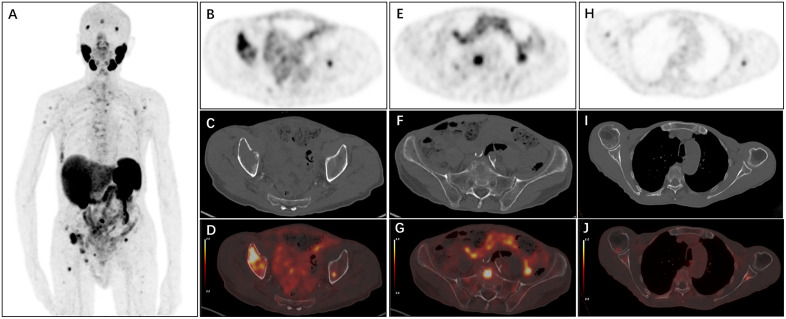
[¹⁸F]PSMA-1007 PET/CT imaging of osseous-only recurrence of prostate cancer following radical prostatectomy. Imaging was acquired in a 62-year-old male with biochemical recurrence (prostate-specific antigen [PSA] = 1.2 ng/mL) at 22 months post-prostatectomy; the primary tumor was graded as Gleason score 7 (ISUP Grade 3). (A) Whole-body maximum-intensity projection (MIP) of [¹⁸F]PSMA-1007 PET demonstrates multiple [¹⁸F]PSMA-1007-avid lesions distributed throughout the skeletal system. Axial PET (B, E, H), CT (C, F, I), and fused PET/CT (D, G, J) images confirm osseous metastases in the bilateral iliac bones (B–D), sacrum (E–G), and left scapula (H–J).
